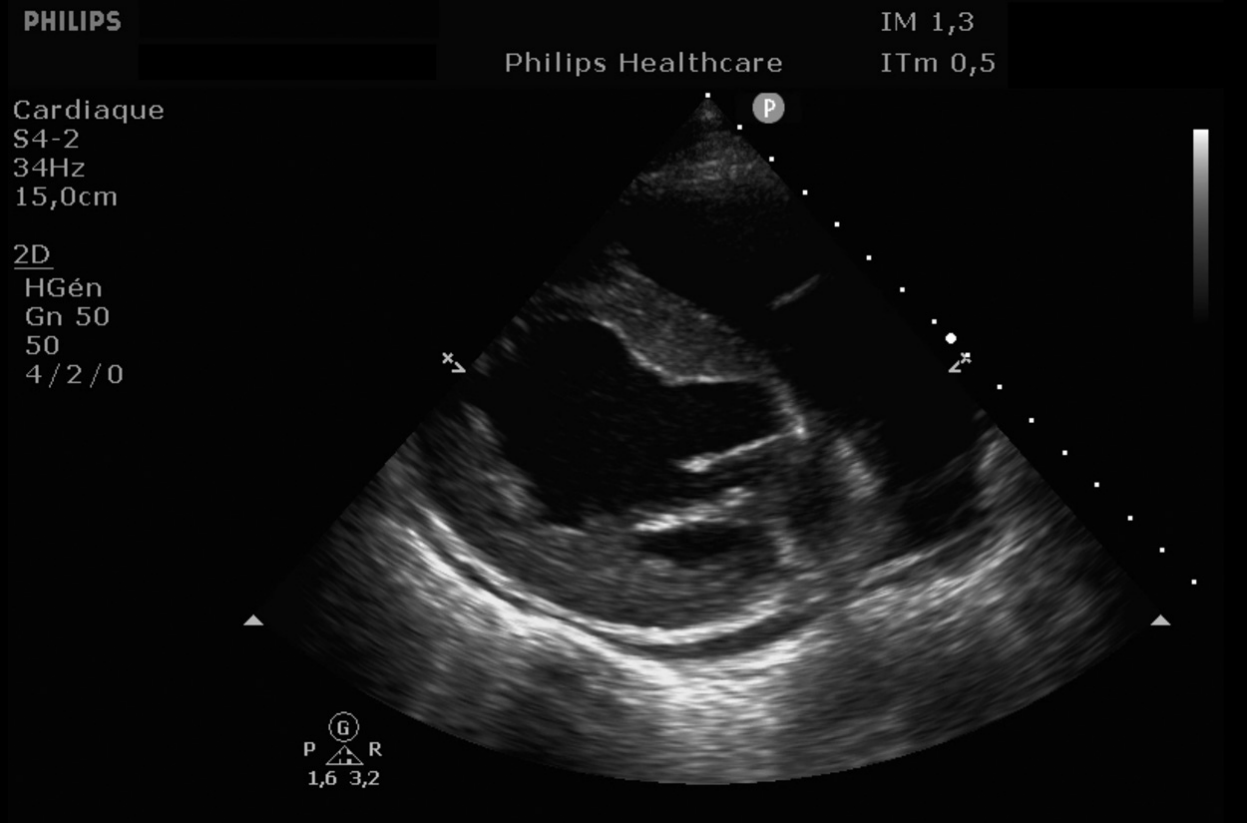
Parasternal long axis of the heart
